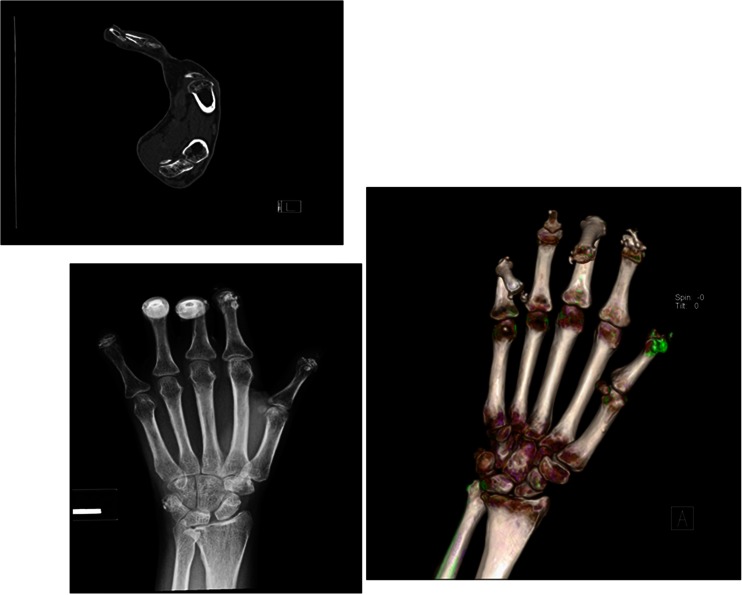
AP view of right hand reveals flexion deformities, acro-osteolysis, and calcinosis of all five fingers. A representative sagittal CT image with bone windows and 3-D volume rendered CT of the third finger shows a clearer example of the findings
